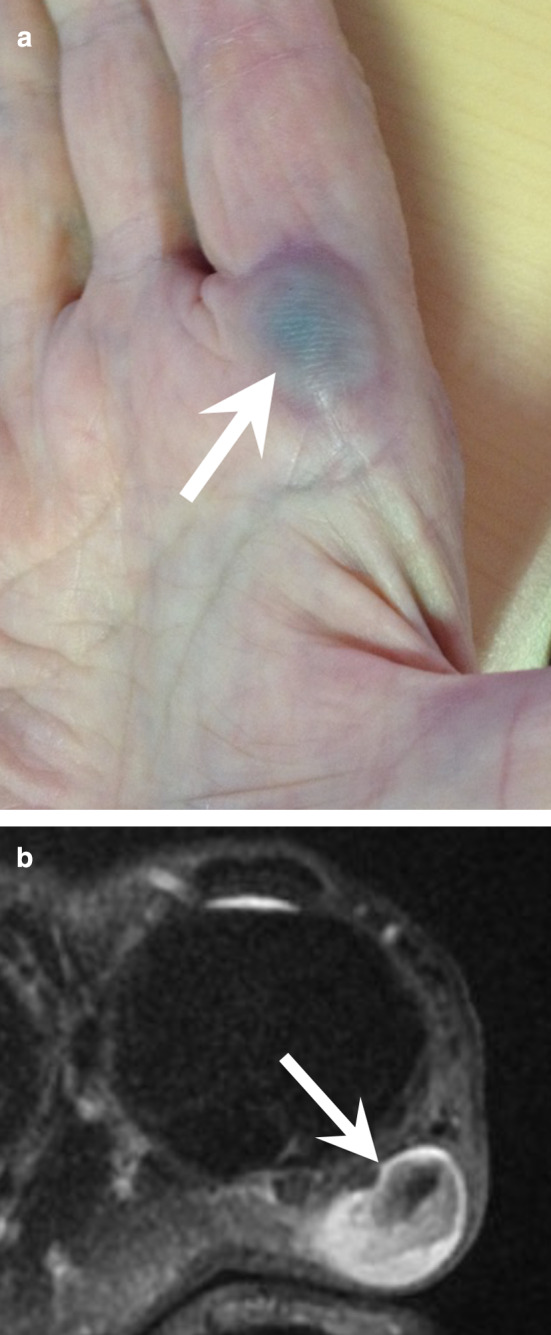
Occluded pseudoaneurysm of the radiopalmar artery of the index finger in a 79-year-old woman with repetitive microtrauma. a Photograph showing a blue mass under the skin at the level of the metacarpophalangeal joint (arrow). b Axial T2-weighted MR image showing the pseudoaneurysm (arrow) with an intraluminal thrombus
